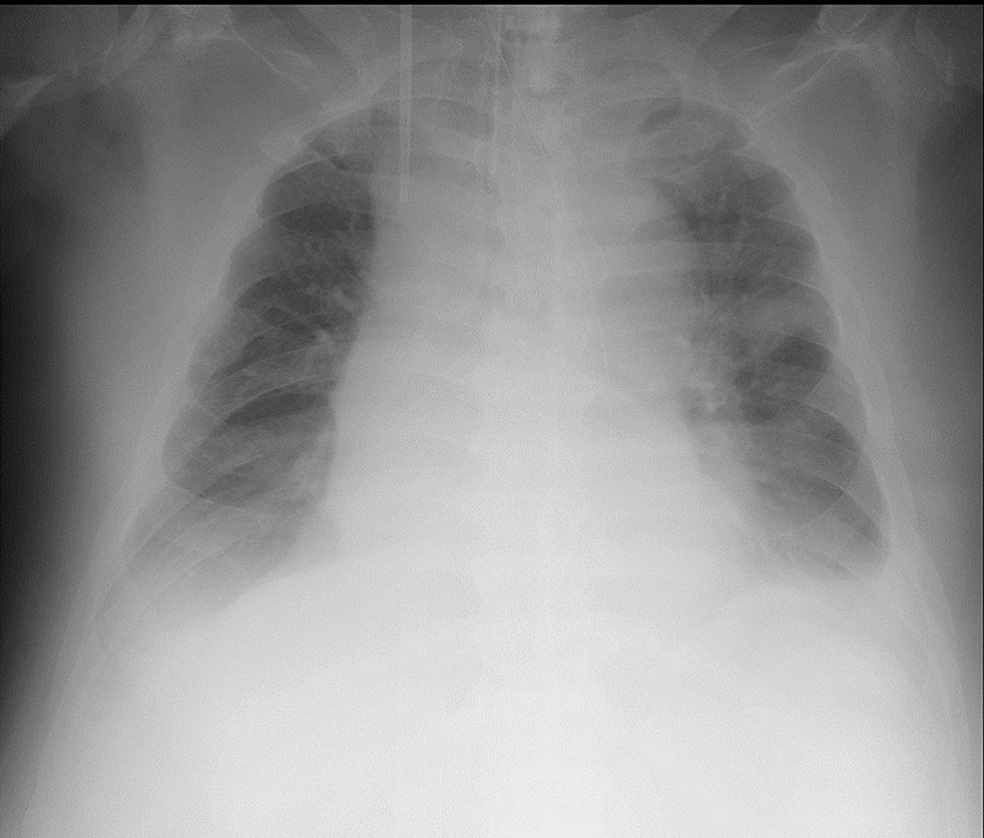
Chest X-ray on the 13th hospital day (after CNAP therapy) The image shows improved atelectasis. CNAP: Continuous negative abdominal pressure
